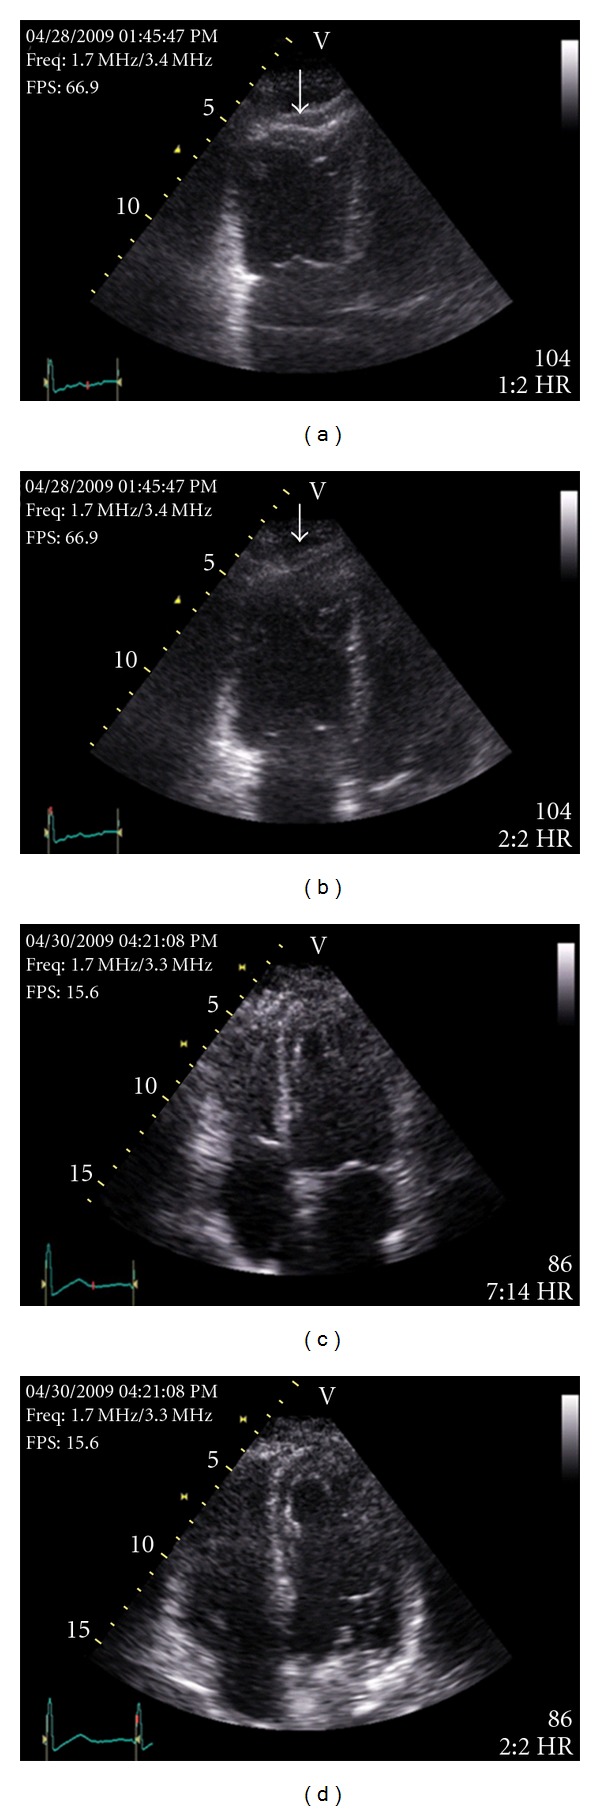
Prethrombolytic therapy transthoracic 2D echocardiogram showing marked hypokinesia of the right ventricle mid-free wall in end-systole (a) and end-diastole (b) while the right ventricle apex is spared (arrows). Postthrombolytic therapy transthoracic 2D echocardiogram showing complete resolution of right ventricular mid-free wall hypokinesia in end-systole (c) and end-diastole (d).
